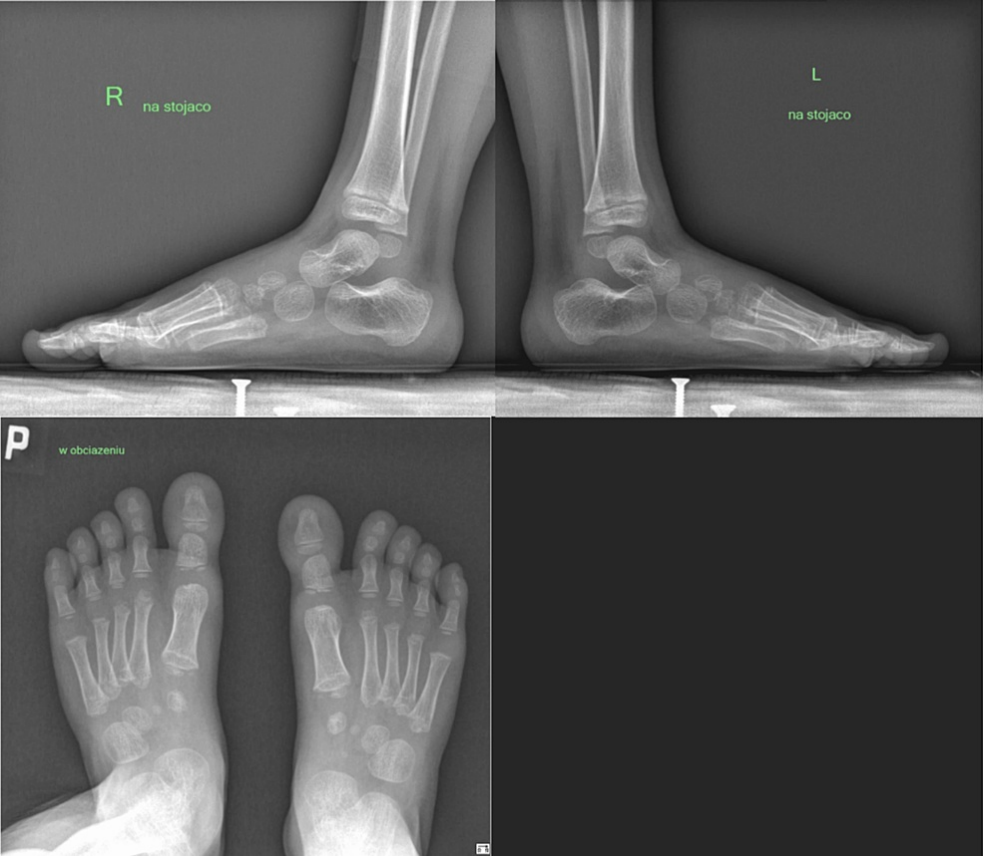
Lateral and standing anterior-posterior (AP) X-rays of the feet post-treatment showing normal bone structure and quality
